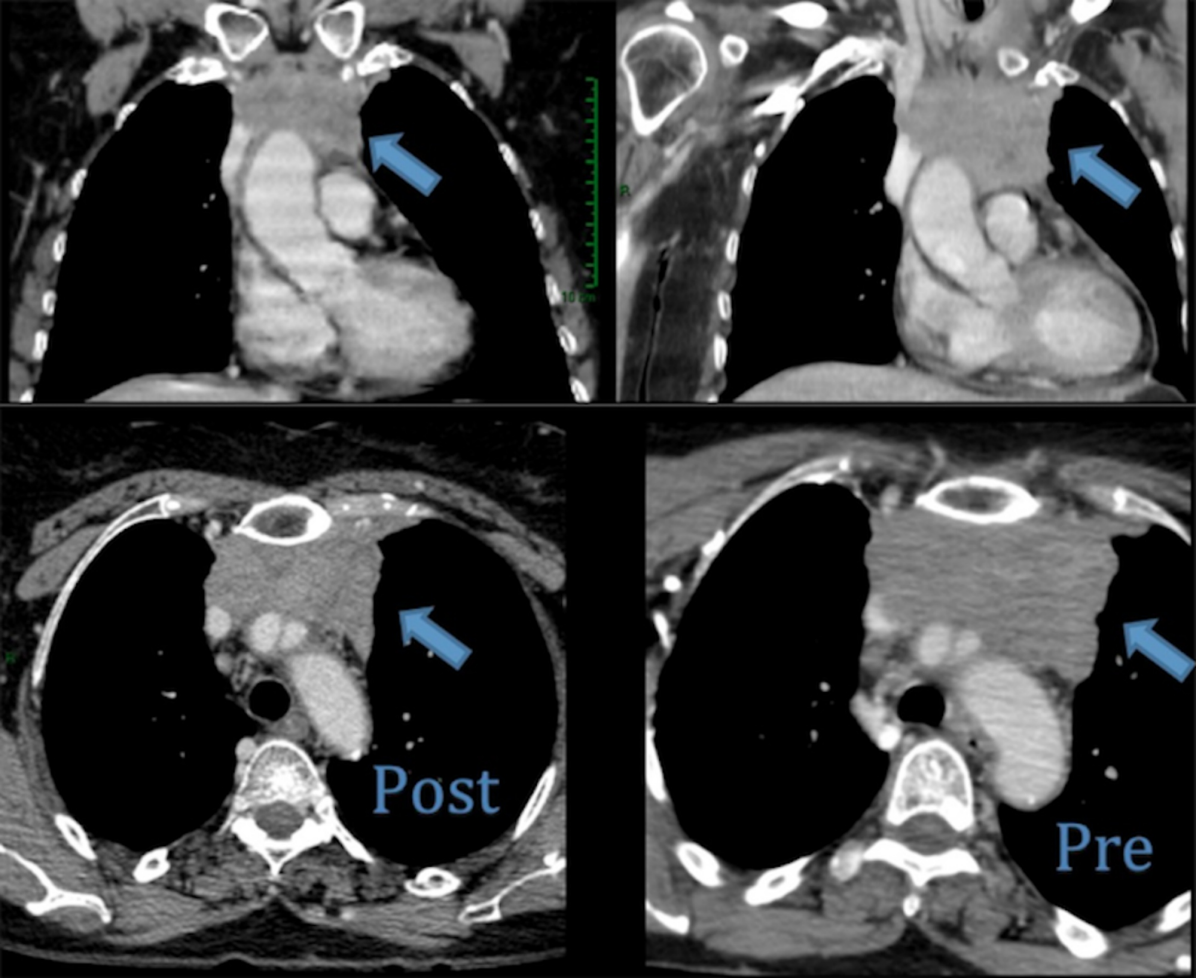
CT scan No significant changes were observed with respect to the previous study, with only a slight decrease in the number of supraclavicular lymph nodes and the size of the mediastinal mass. In the right part of the image (Pre), the thymic carcinoma is shown before the chemotherapy treatment, and in the left part (Post), it is shown after the treatment. CT: computed tomography
